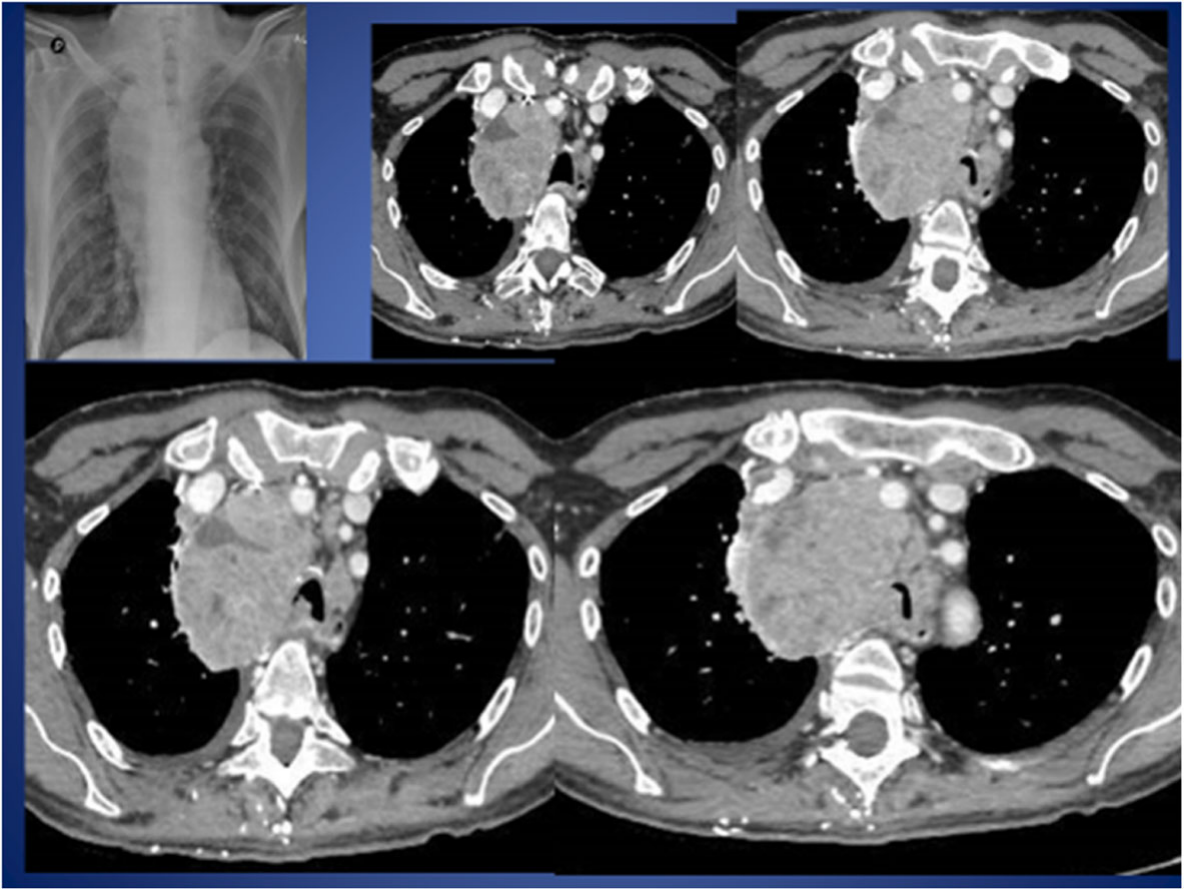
Chest radiography and Computed Tomography (CT) showed a solid neoplastic lesion in the apical segment of the right lower lobe and significant stenosis of the tracheal lumen
